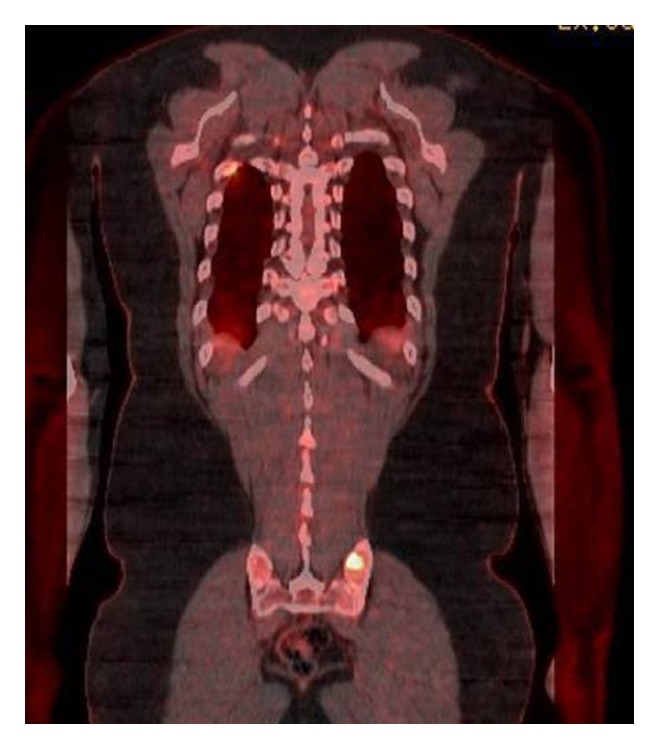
FDG avid lesion in left sacrum.
